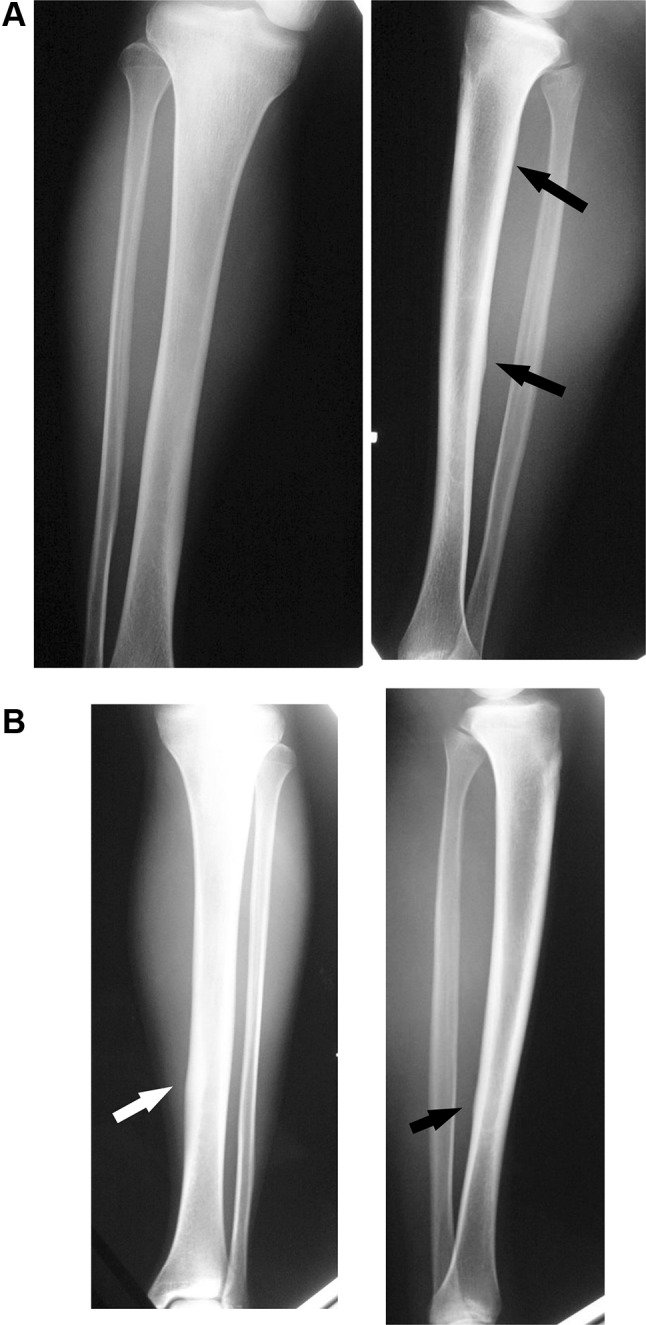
Radiographs of both legs 6 months after the initial visit to our hospital. Obtained 6 months after the first examination, callus formation is seen at the lateral and posterior side of the tibia in the antero-posterior and lateral views. The arrows indicate callus formation at the lateral and posterior sides of the tibia
